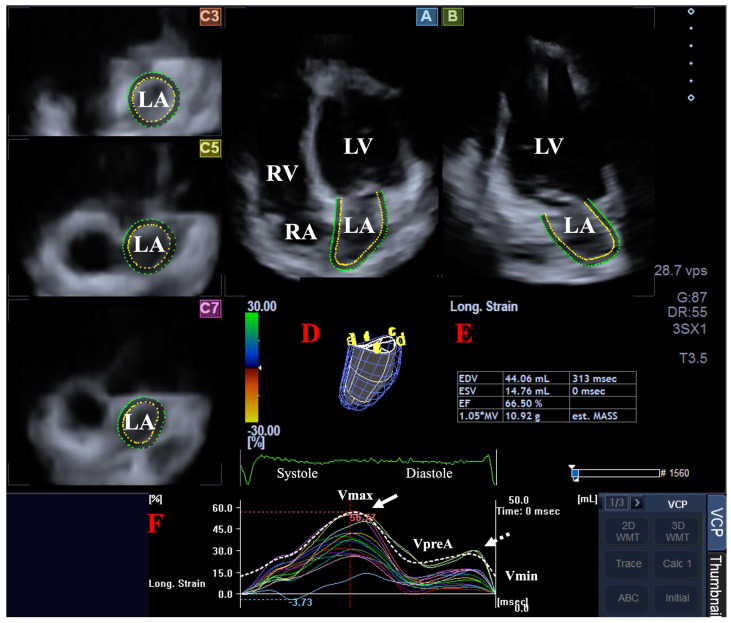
Three-dimensional (3D) speckle-tracking echocardiographic (3DSTE) assessment of the left atrium (LA) in a healthy subject using a full-volume dataset is demonstrated. The analysis incorporates apical four-chamber (A) and two-chamber (B) views, as well as short-axis views at the basal (C3), midatrial (C5), and superior (C7) levels to create a comprehensive 3D LA cast (D). LA volumetric data (E) are presented alongside with time-dependent curves for global LA volume change (represented by a dashed line) and segmental LA longitudinal strains (represented by colored lines) (F). In the visualization, the white arrow indicates peak LA strains, while the white dotted arrow marks LA strains specifically at atrial contraction. Abbreviations. LA, left atrium; LV, left ventricle; RA, right atrium; RV, right ventricle; EDV, end-diastolic volume; ESV, end-systolic volume; EF, ejection fraction; Vmax, maximum LA volume; VpreA, pre-atrial contraction LA volume; Vmin, minimum volume.
